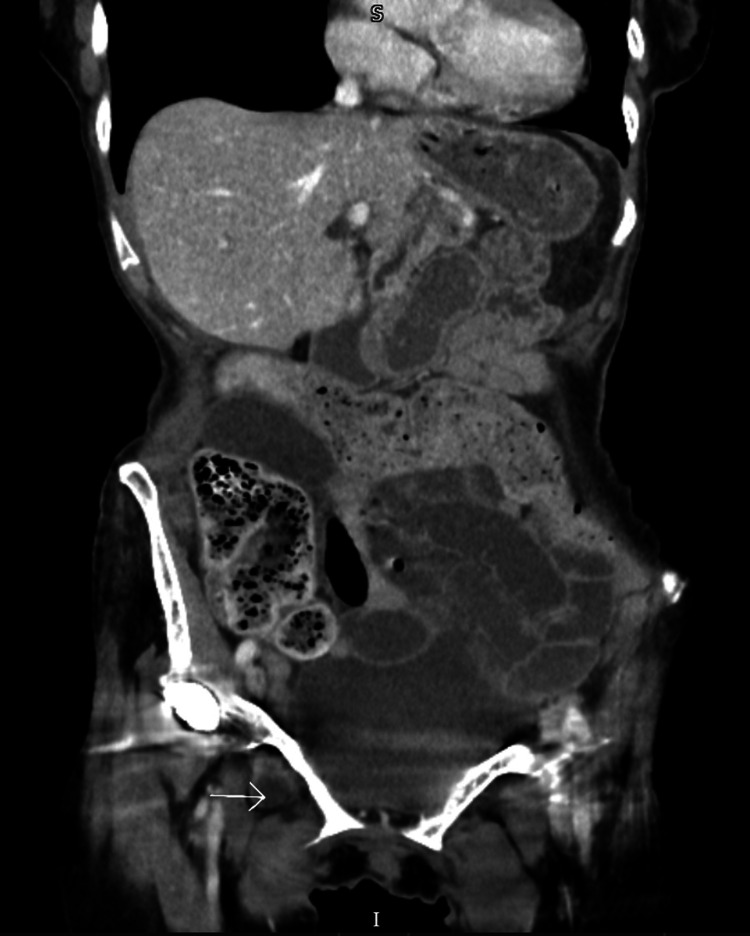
CT scan of abdomen and pelvis (coronal plane) showing the right-sided obturator hernia (arrow). CT: computed tomography
